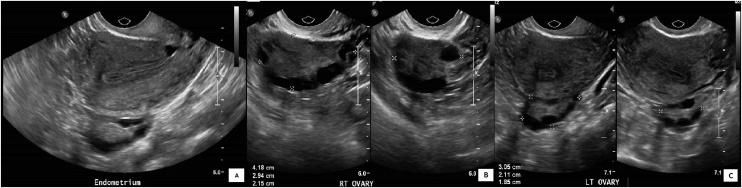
Transvaginal ultrasound pelvis images. [A] Sagittal view of the uterus with normal endometrium thickness [B] Right ovary enlarged with polycystic morphology (vol14cc). [C] Left ovary is normal.
